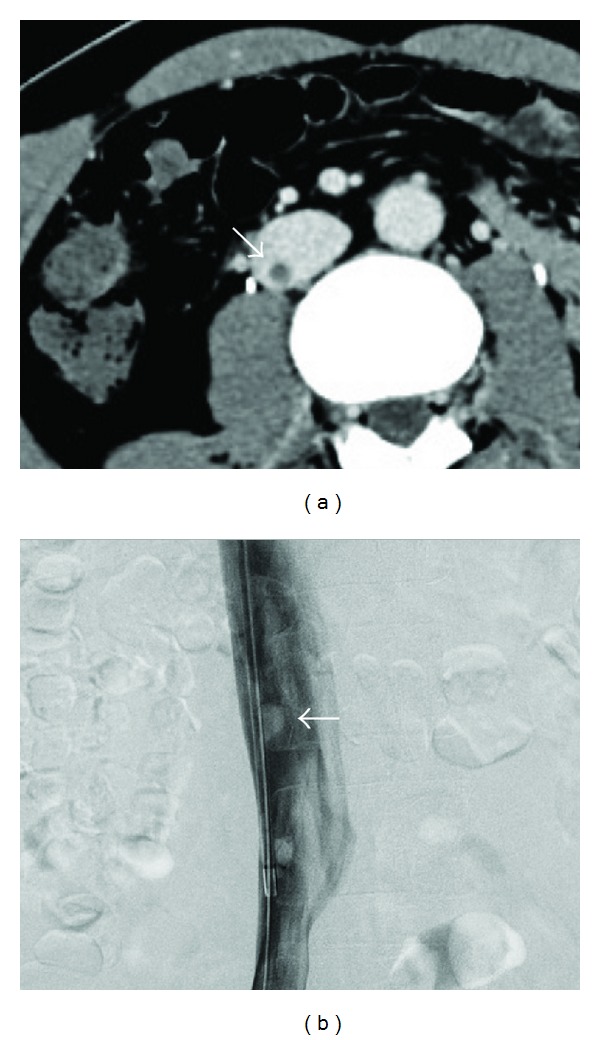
Contrast-enhanced computed tomography (a) and catheter-based venography (b). A small, polyp-like thrombus was observed at the infrarenal inferior vena cava.
